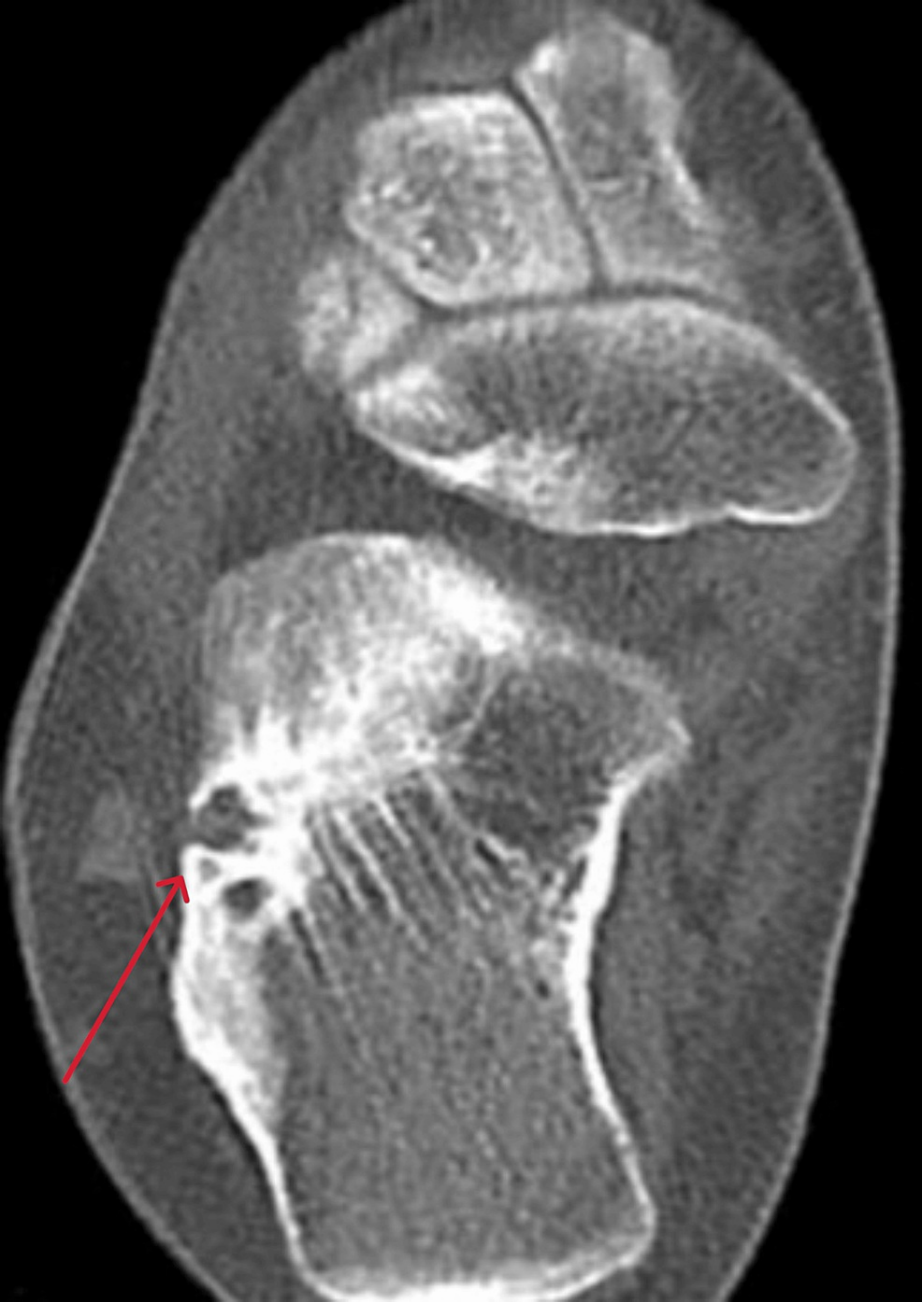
Axial view CT scan of the right ankle at one-and-a-half-year follow-up CT: computed tomography
